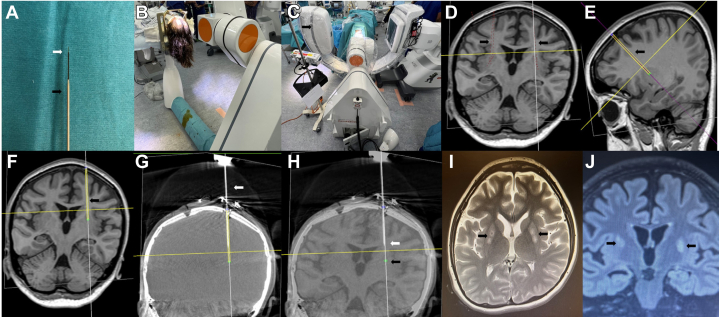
(A) Infusing end of the SmartFlow cannula, showing the termination of the rigid outer silicon cover (black arrow) and the stepped infusion tip (white arrow) which is designed to reduce backflow of infusate along the length of the cannula. (B, C) Operative room set-up for infusion of gene therapy into the putamen in a child with AADCd using the Renishaw robot. (B) The patient, supine, with the head immobilized to the robot in a Leksell stereotactic frame. (C) The patient and the robot arm are draped, and the mobile intraoperative 3-dimensional C-arm is positioned to allow intermittent imaging for verification of cannula trajectory. (D) T1-weighted MRI scan showing the right and left, anterior and posterior trajectories as planned on the NeuroInspire software (black arrows); the robot arm is aligned to the left anterior trajectory. (E, F) T1-weighted MRI scans in (E) sagittal and (F) trajectory planes (black arrows) to the left anterior putamen. (G) Intraoperative mobile computed tomography scan after insertion of SmartFlow cannula along the left anterior trajectory (white arrow); (H) when fused to the preoperative MRI scan, the trajectory of the cannula along the preplanned trajectory is verified (white arrow). The visible cannula falls short of the target (black arrow) as the length of the cannula beyond the silicon outer cover is radiolucent. (I, J) Intraoperative MRI scan after final injection and withdrawal of the cannula, (I) T2-weighted and (J) fluid-attenuated inversion recovery (FLAIR), confirming visible satisfactory infusion of therapeutic agent without coinfusion of gadolinium.
